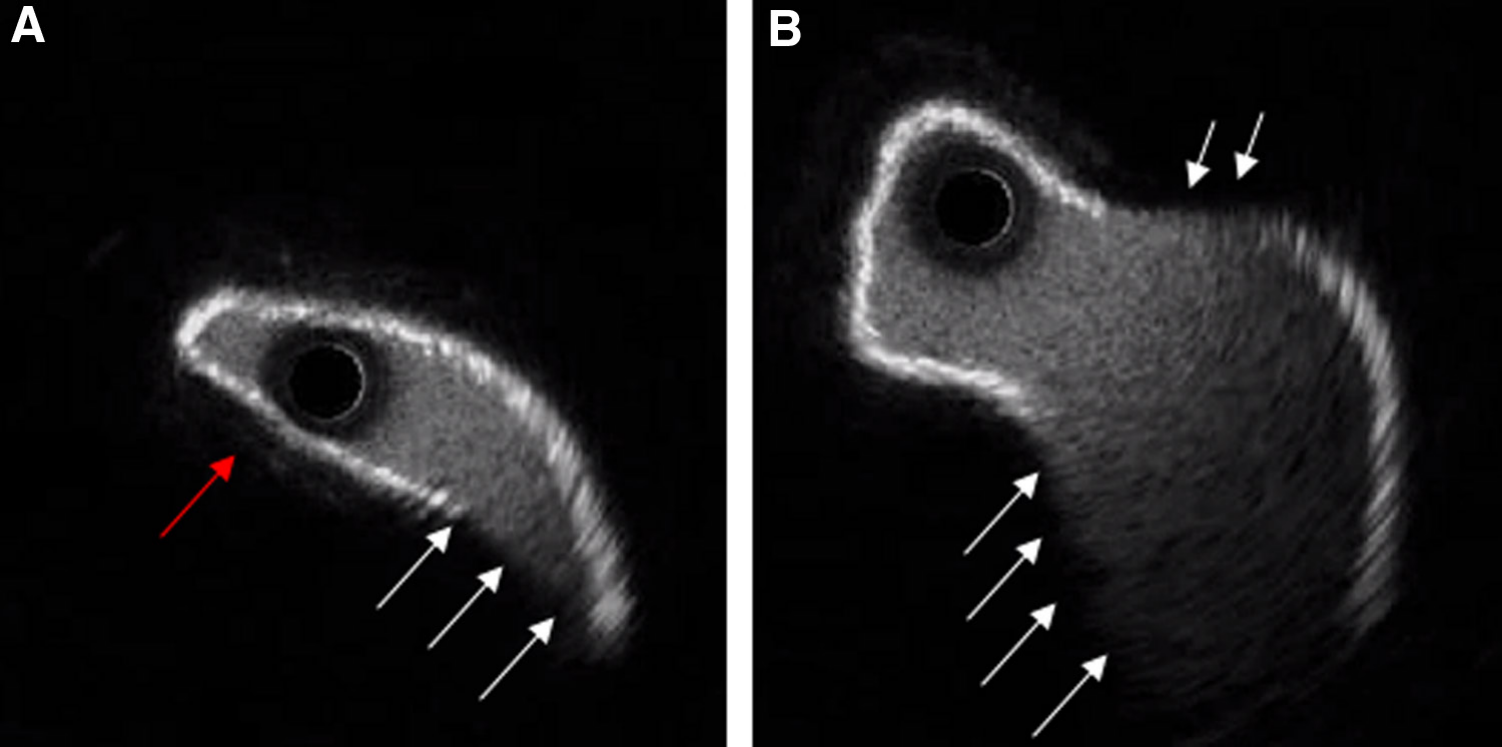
IVUS assessment of the outflow graft. A and B: IVUS before stenting demonstrates an oval-shaped luminal narrowing caused mainly by external compression (red arrow), but also some degree of torsion as indicated by the echolucent luminal borders (white arrows) as a result of twisting in the acoustic shadow of luminal borders that are in closer proximity to the IVUS catheter. IVUS, intravascular ultrasound.
